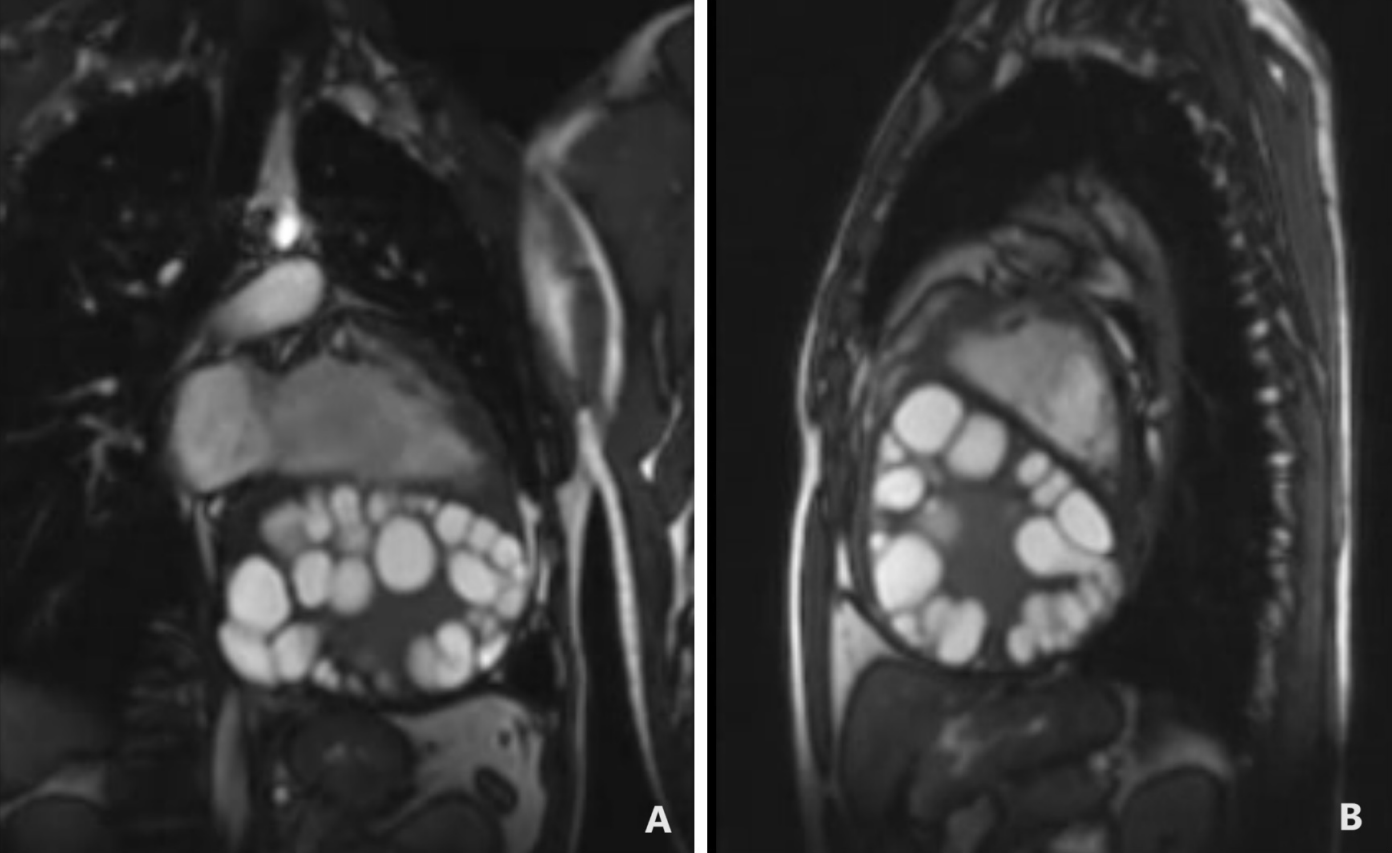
Cardiac magnetic resonance imaging through static images of a cine loop. (A) The sagittal section features a four-chamber view, while (B) displays a short-axis view. Both images depict multiple hypointense ring-shaped thick-walled hydatid cysts occupying the entire cavity of the left atrium and left ventricle.
